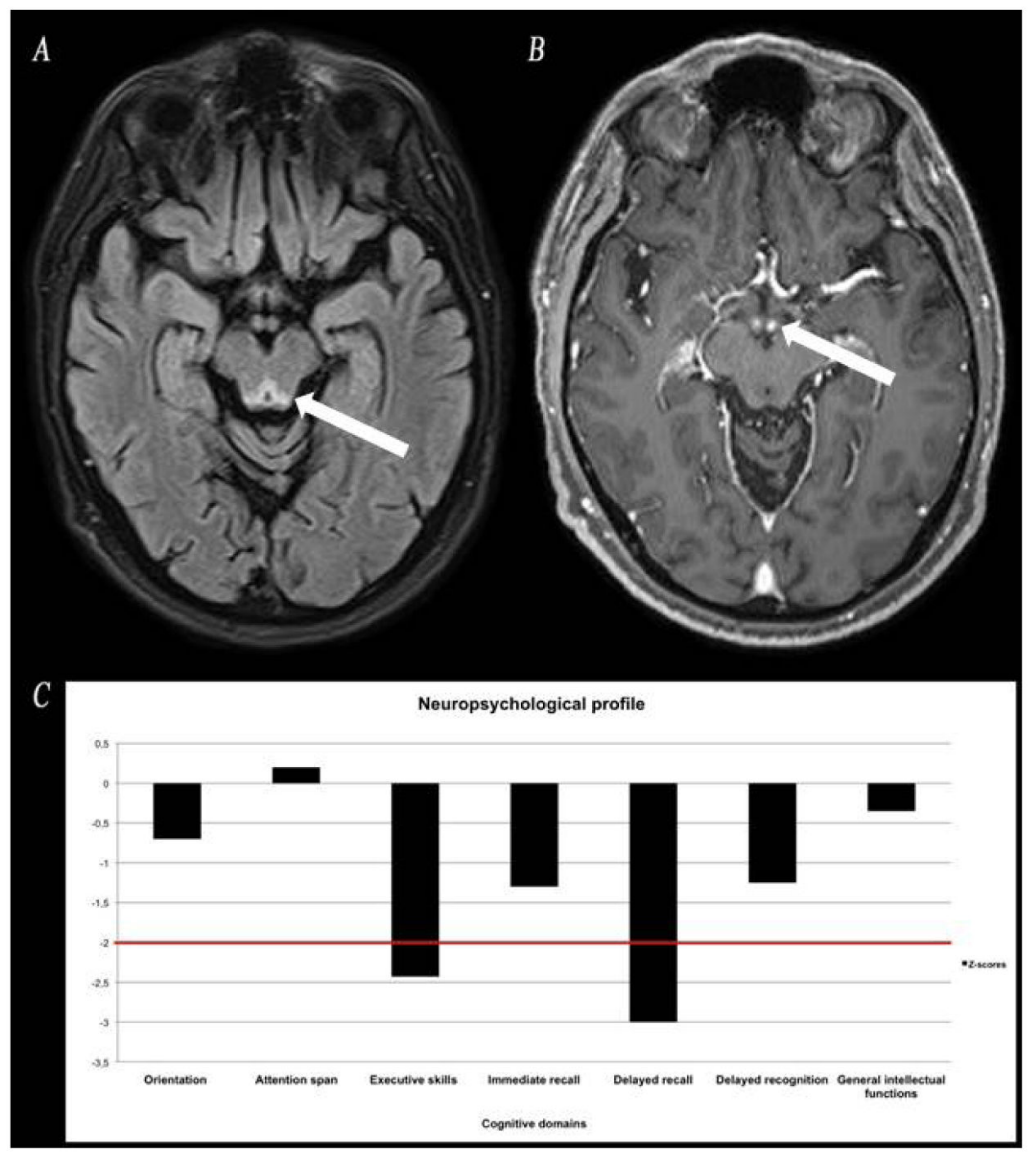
A. MRI of the brain showing increased T2/FLAIR in periaqueductal area. B. A 2nd MRI performed seven days after initial study revealed bilateral enhancement of the mammillary bodies on T1/gadolinium sequence. C. Neuropsychological assessment conducted two weeks after admission documented significant deficits in memory retrieval processes and executive skills.
